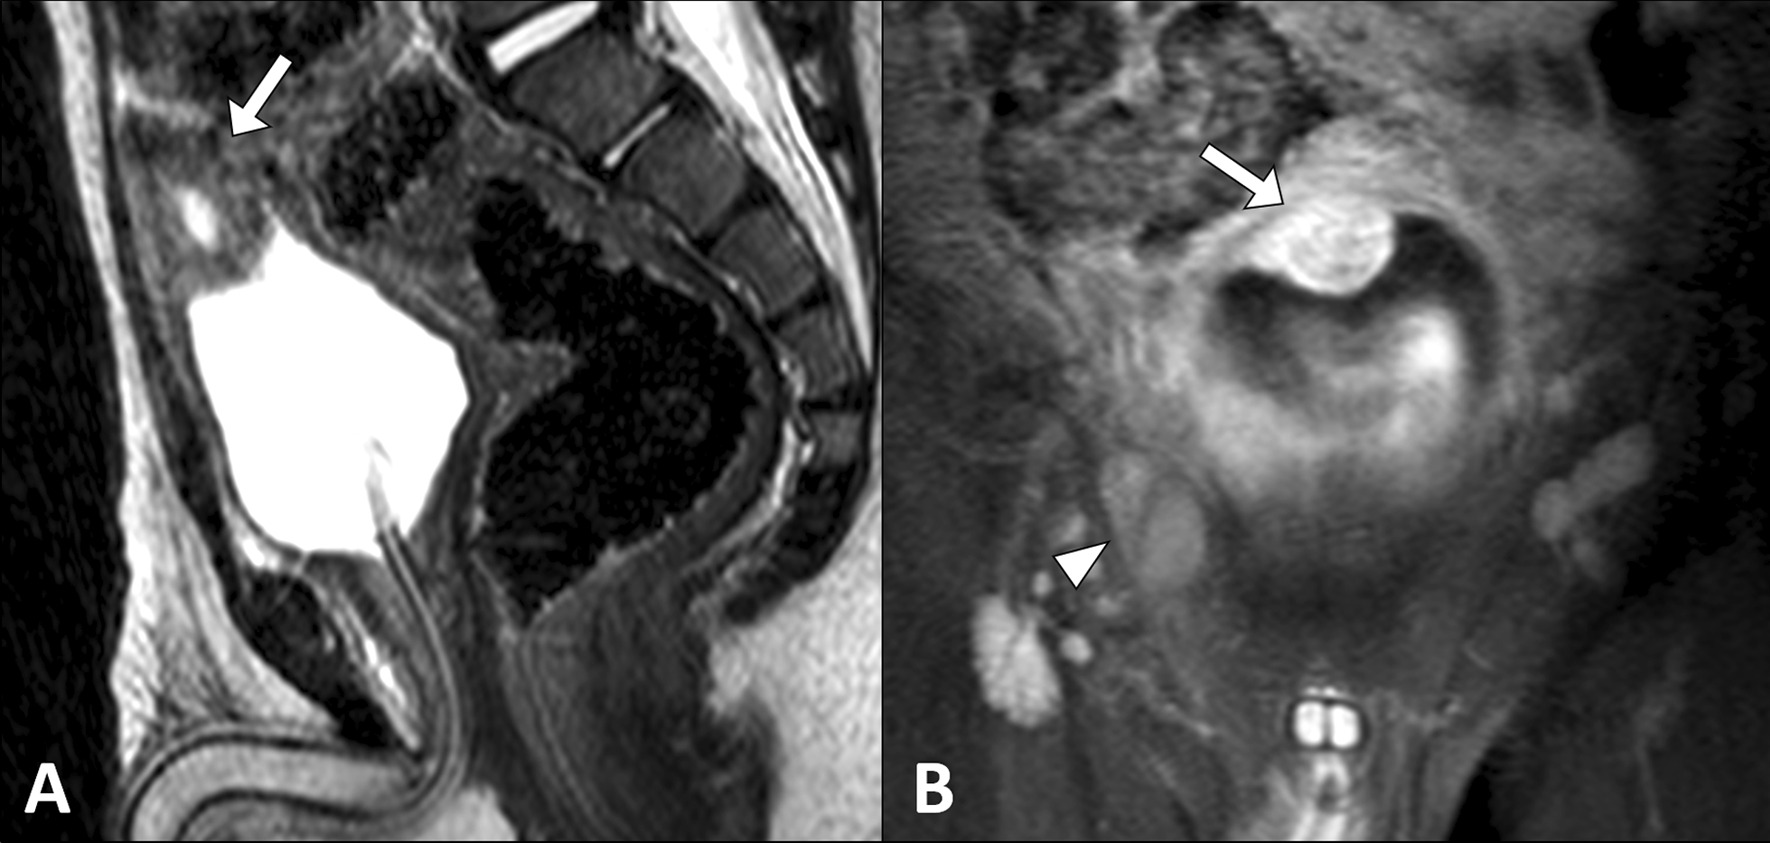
T2-weighted magnetic resonance image (A) shows a cystic lesion (arrows) arising from the anterosuperior wall of the urinary bladder and protruding into its lumen. Coronal, post-contrast, fat-suppressed, T1-weighted image (B) shows heterogeneous enhancement of the lesion, with an incidental finding of retractile right testis in the inguinal canal (arrowhead)
